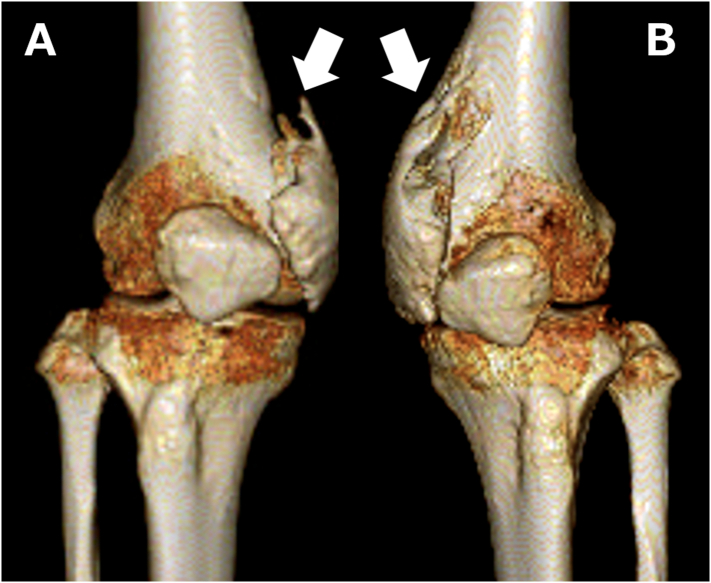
Three-dimensional computed tomography of the knees showing heterotopic ossification on the peripheral areas of knee joints, especially on the medial compartment (arrow). A: Three-dimensional computed tomography of the right knees B: Three-dimensional computed tomography of the left knees.
